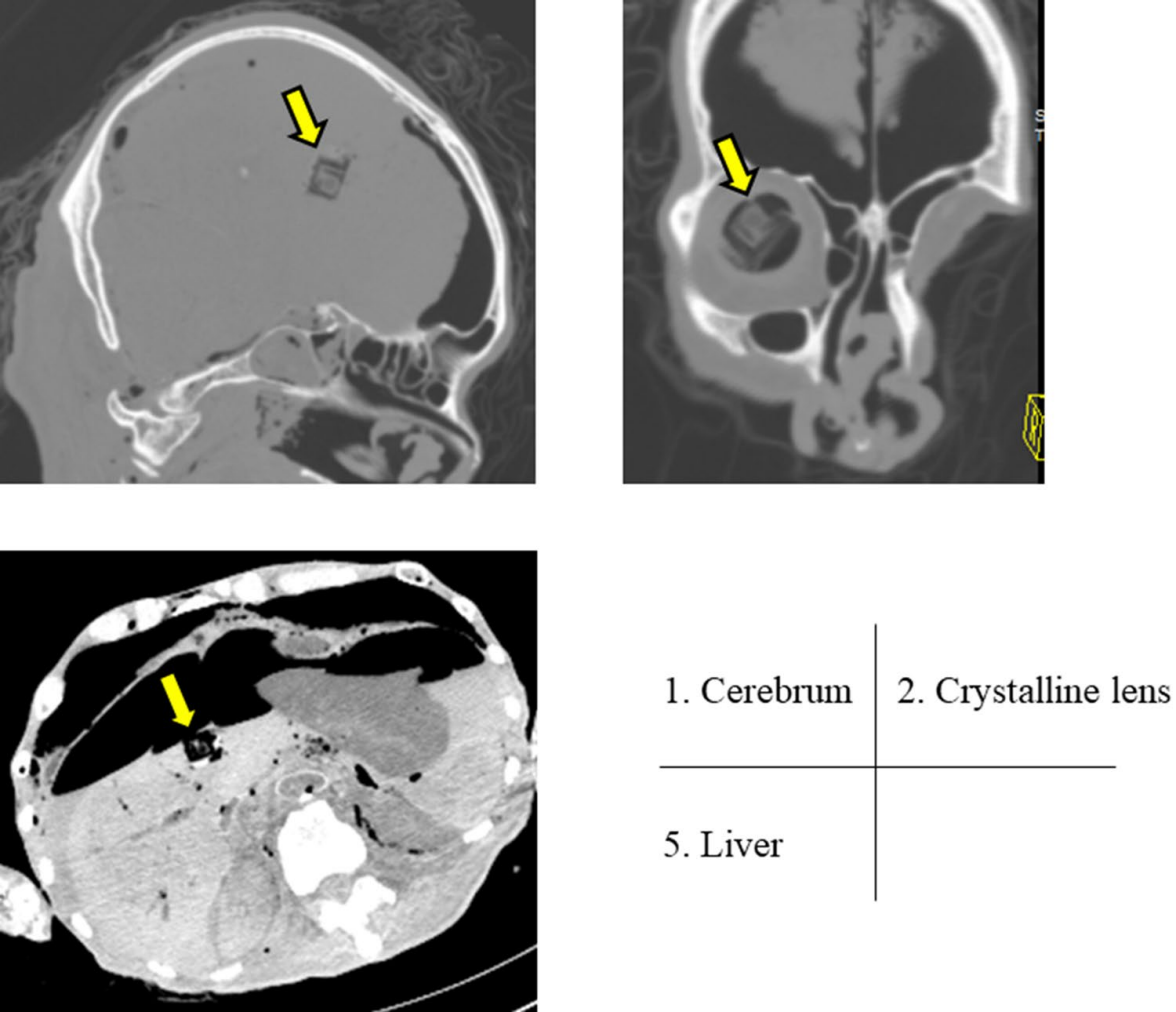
CT imaging of individual organs. CT image showing that all dosimeters (arrow) were placed at appropriate positions and did not affect the image.
